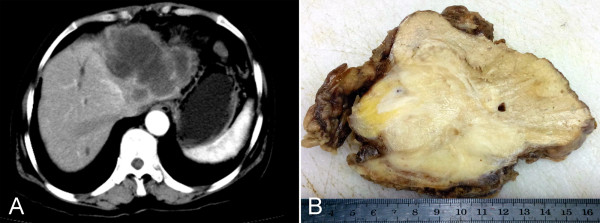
Preoperative and gross findings of tumor. (A) Computed tomography (CT) showed a large tumor lesion, 8.0 cm in diameter, in the left lobe of the liver. (B) Gross examination of resected liver mass showed an irregular, yellowish white solid tumor without a fibrous capsule. The border between the tumor and normal liver tissue was indistinct.
